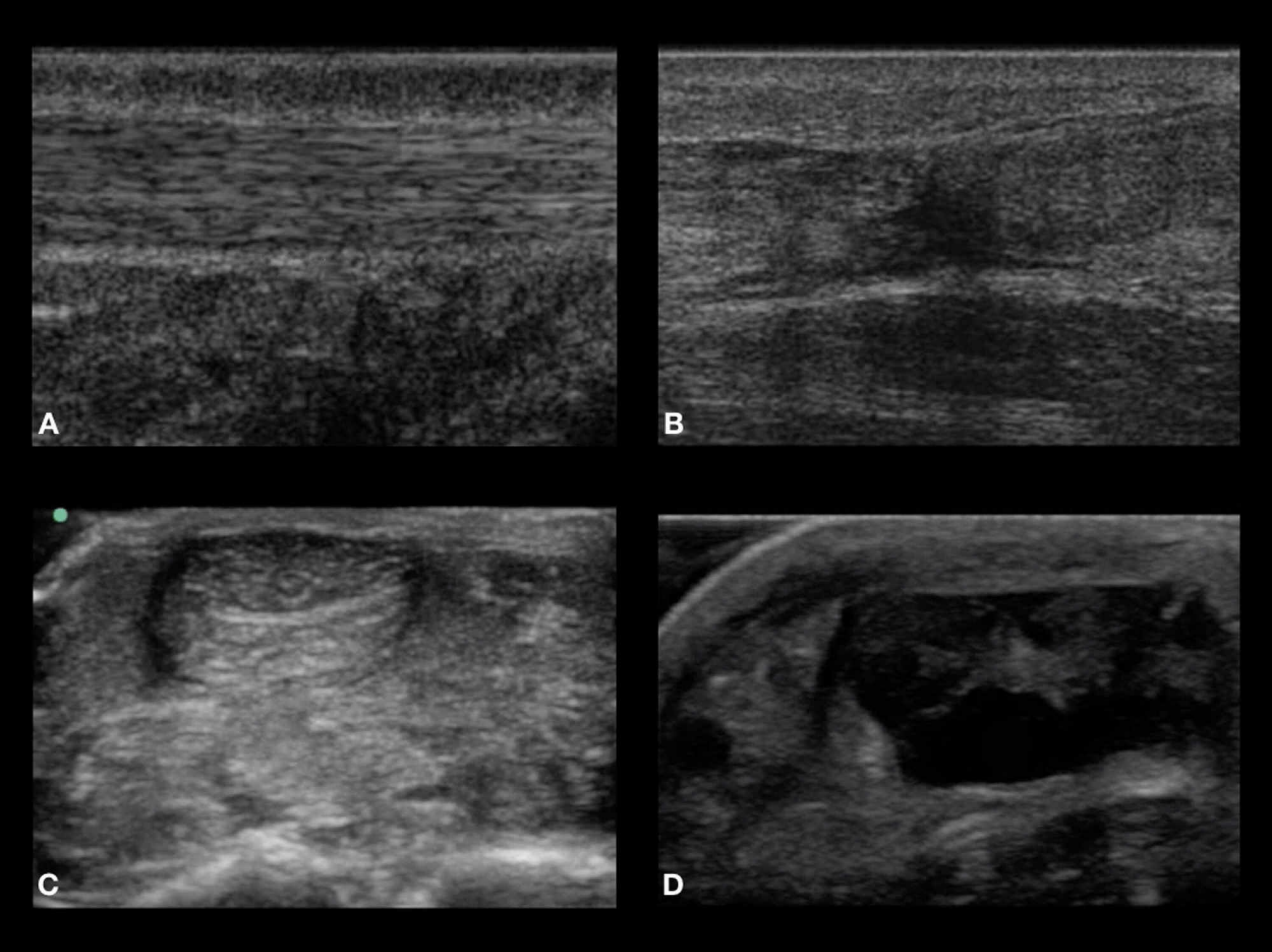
Panels A and C represent the normal sonographic Achilles tendon appearance in the sagittal and transverse planes, respectively. Panels B and D represent ruptured Achilles tendons seen in the sagittal and transverse planes, respectively. All images obtained were from actual cadavers used.
